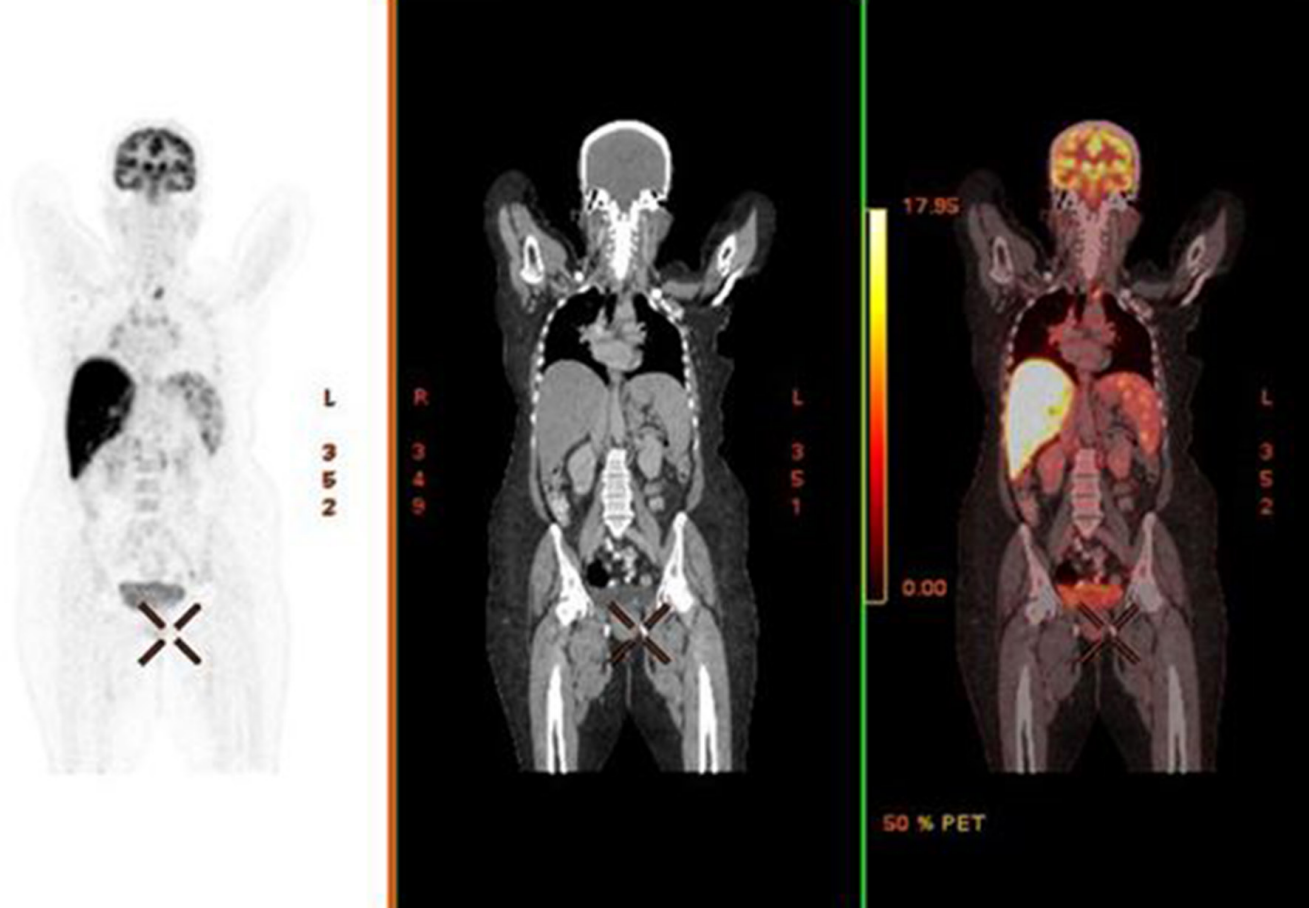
Coronal PET, CT and corresponding fused PET/CT image showing hypermetabolic/hot liver. PET image obtained one hour after administration of 5.2 mCi of ^18^F-FDG. CT done without administering intravenous contrast. FDG, fluorodeoxyglucose; PET, positron emission tomography.
